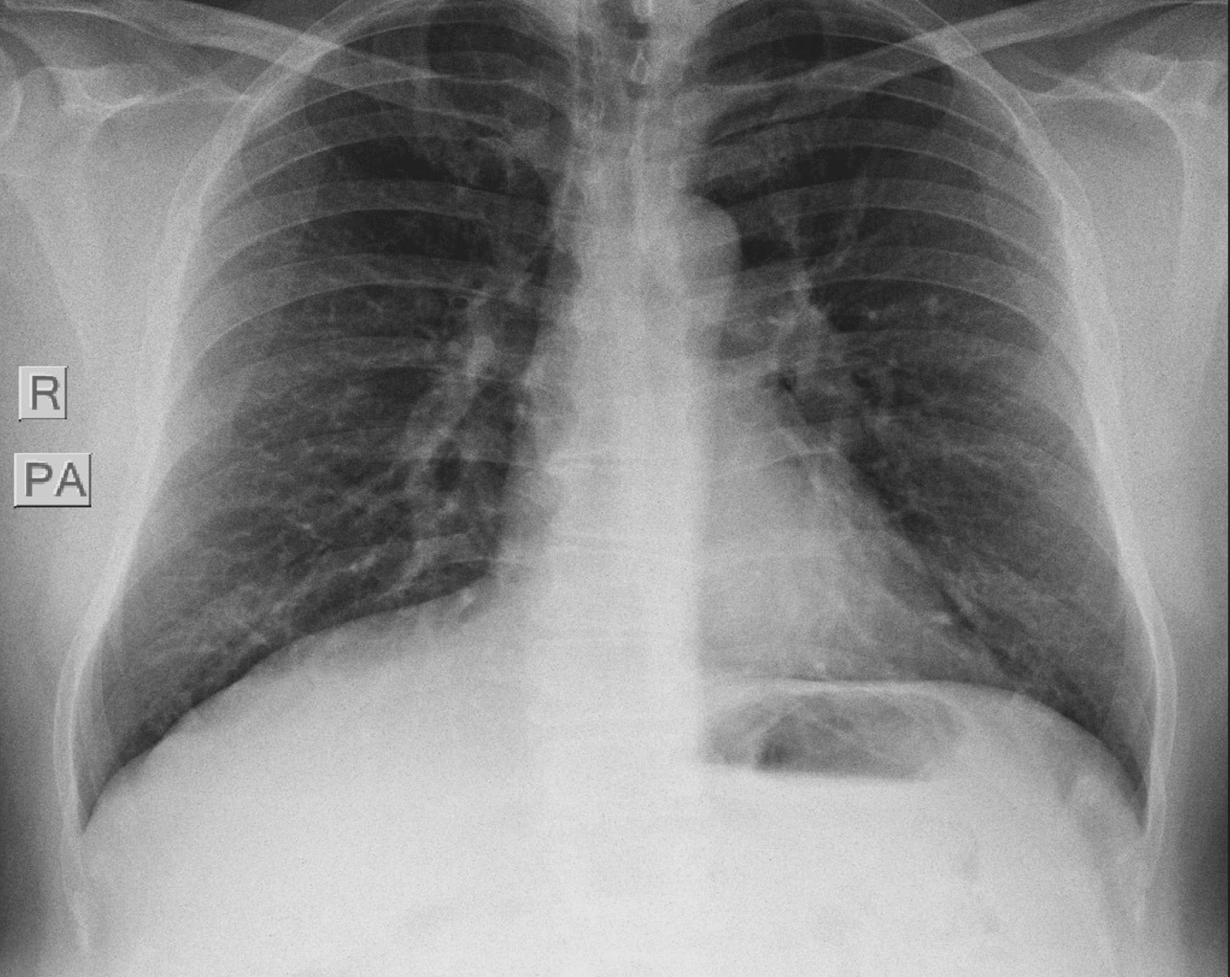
Grossly normal chest X-ray
